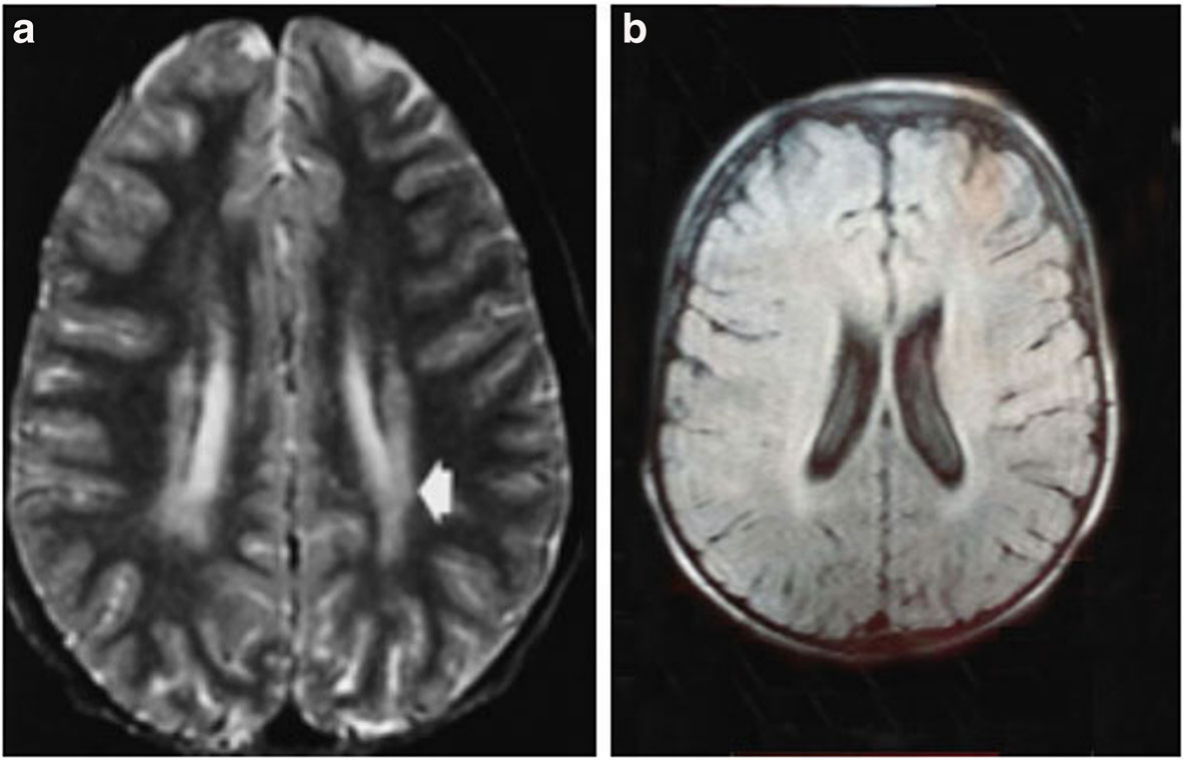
MRI report of patient I. a mild abnormal signal changes in periventricular white matter, b mild abnormal signal changes in periventricular white matter in T1 sequence
